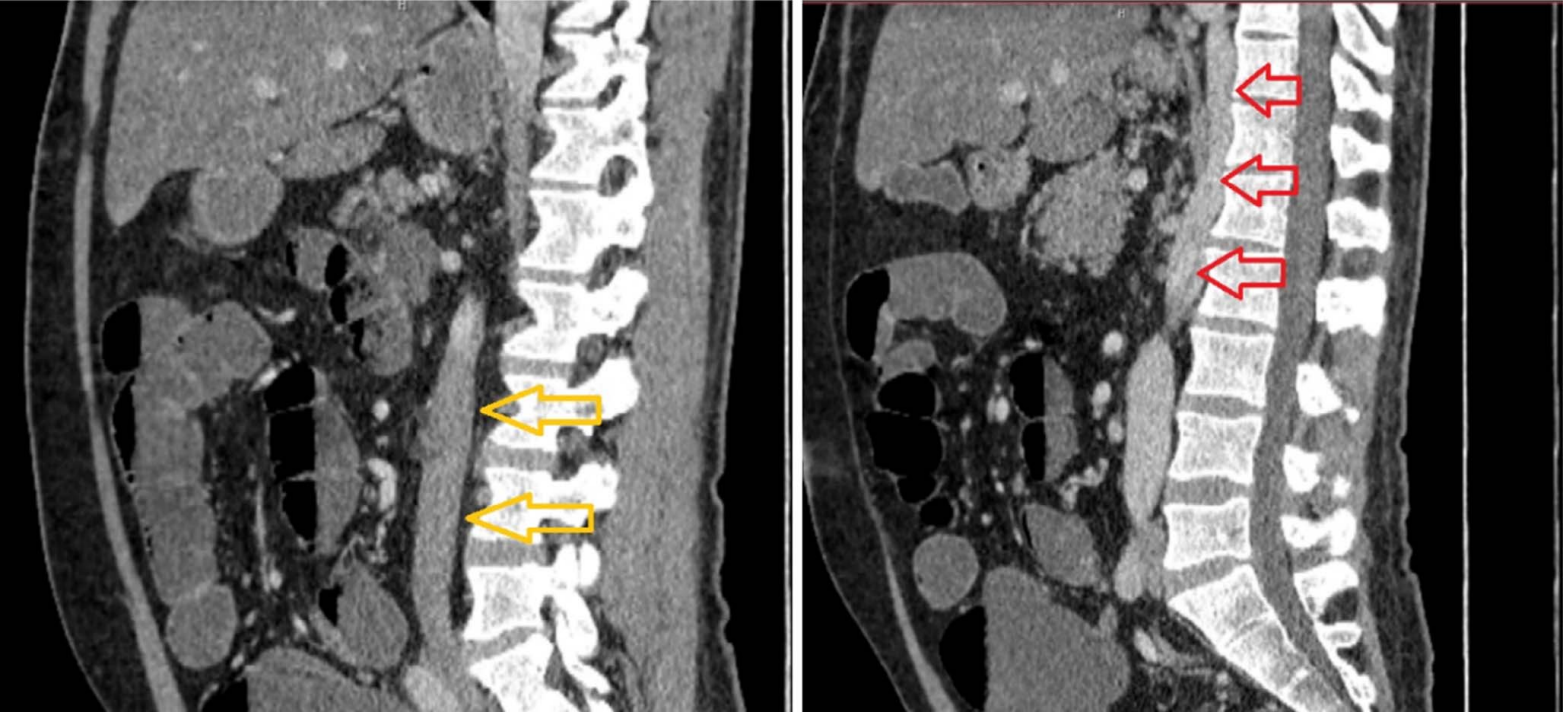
Consecutive sagittal plane computed tomography images show (left image) the interruption of the vena cava (yellow arrows) at the suprarenal level and (right image) continuity with the dilated azygos vein (red arrows).
